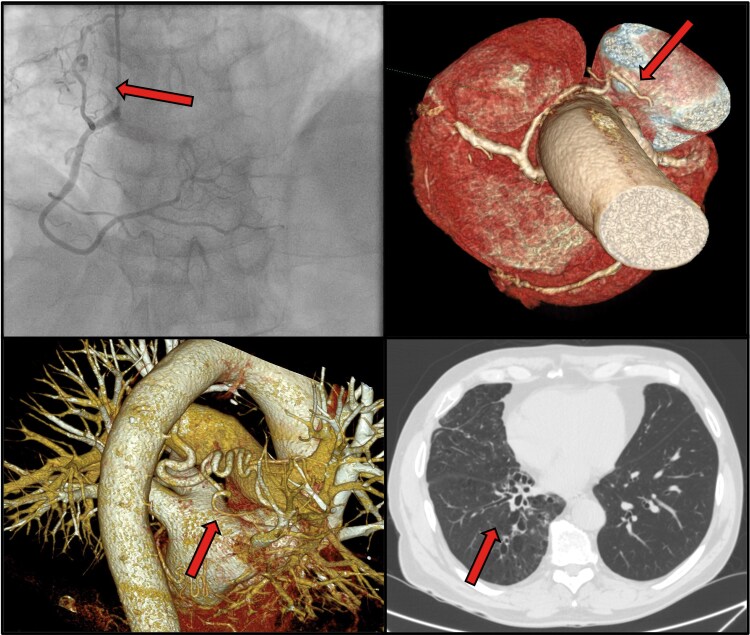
Multimodality imaging of a coronary–bronchial artery fistula. Upper left: invasive coronary angiography of the right coronary artery. The arrow indicates a hypertrophied atrial branch arising from the proximal segment and coursing posteriorly. Opacification of the right pulmonary parenchyma after contrast injection suggests communication with pulmonary or bronchial collateral vessels. Upper right: volume-rendered coronary computed tomography angiography. An atrial branch with a retroaortic course is visualized, showing distal bifurcation. The arrow indicates the branch converging with the bronchial artery along the superior aspect of the left atrium. Lower left: volume-rendered coronary computed tomography angiography demonstrating a hypertrophied bronchial artery originating from the anterior surface of the descending thoracic aorta and following a tortuous course towards the right bronchial tree. The arrow indicates the convergence between the atrial branch and the hypertrophied bronchial artery. Lower right: chest computed tomography showing cylindrical and varicose bronchiectasis in the right middle and lower lobes, with mucous plugging and radiological findings suggestive of superinfection.
